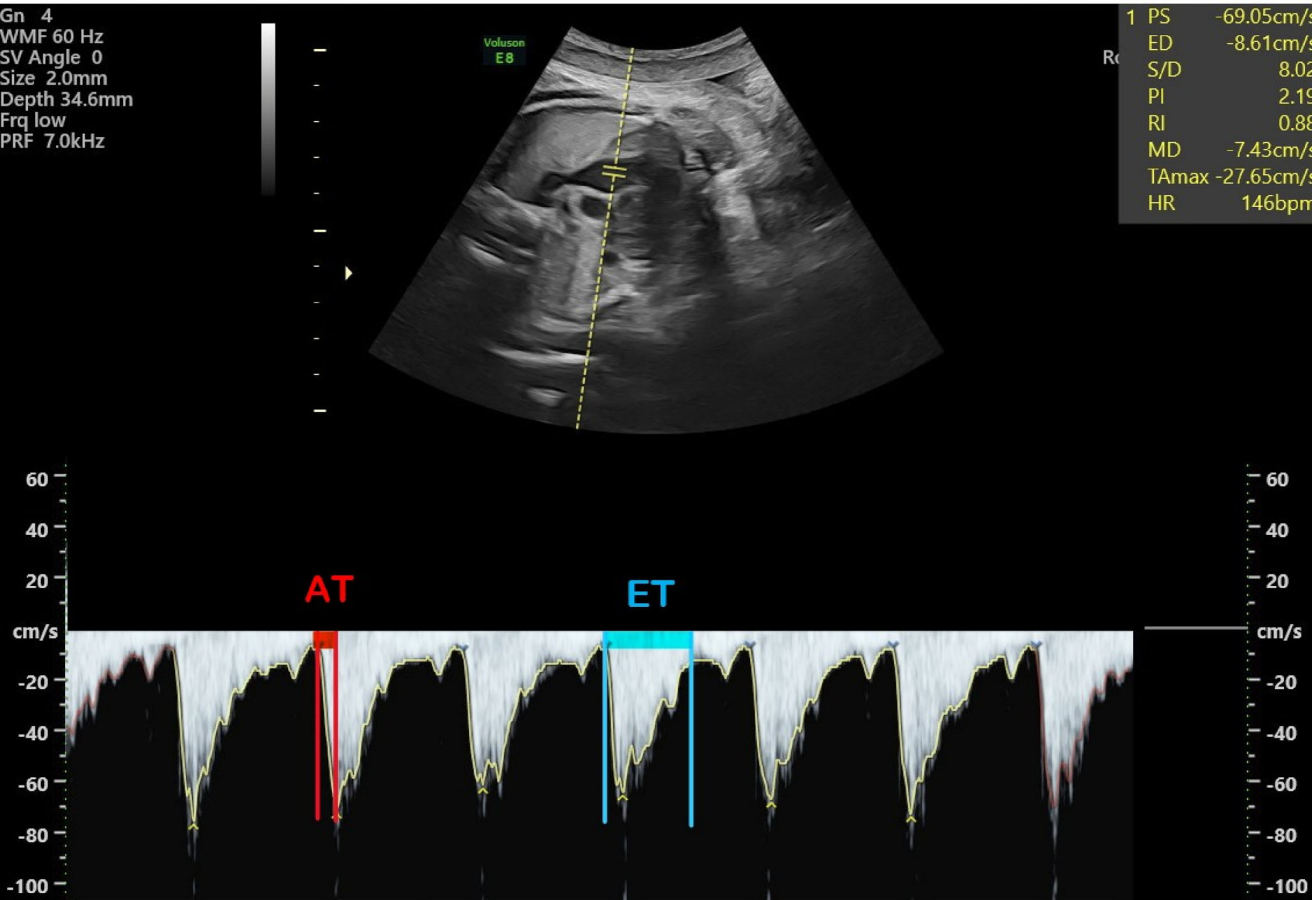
Main pulmonary artery Doppler parameter. AT stands for acceleration time, which is the time between the start of systole and its peak, and ET stands for ejection time, which is the time between the start of systole and its end.
